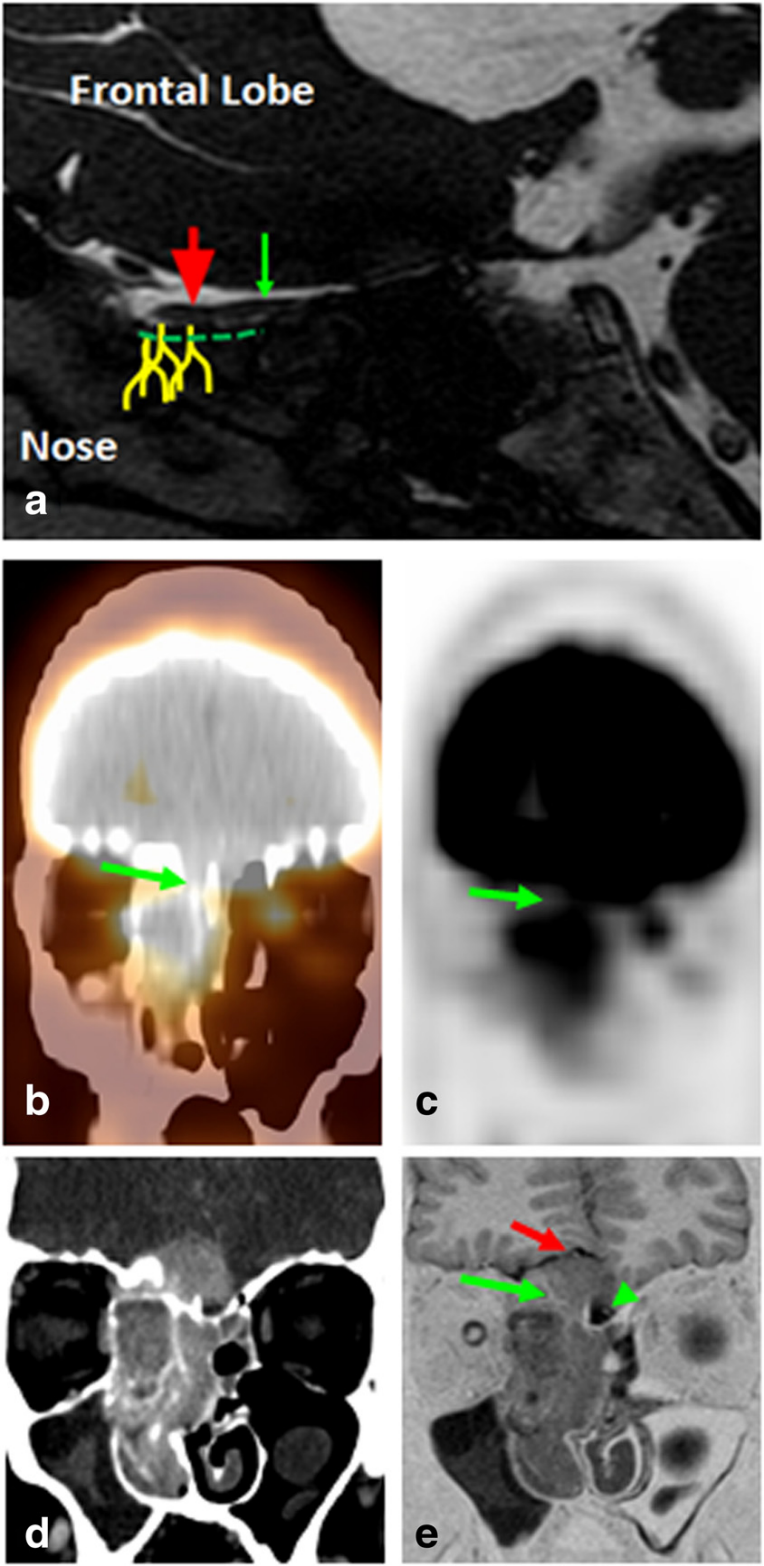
a Sagittal steady state free precession (SSFP) MRI image of the brain showing the olfactory nerve anatomy: The fibers in the superior nasal mucosa (yellow fibers), ascend through the fenestrated cribriform plate of the ethmoid bone (green dashed line) to reach the anteriorcranial fossa and continue as the olfactory bulb (red arrow) and tract (green arrow) coursing atthe inferior surface of the ipsilateral frontal lobe. b–e A 38-year-old male presented with a 6month history of anosmia and episodic epiphoresis, nasal stuffiness. Coronal fused (a) andunfused (b) PET/CT images demonstrate a large aggressive hypermetabolic mass centered on thesuperior aspect of the nasal cavity, extending superiorly into the right anterior cranial fossa andcribriform plate of ethmoid bone, involving the expected location of the right olfactorynerve/bulb/track (arrow). Our differential diagnosis was olfactory neuroblastoma (Esthesioneuroblastoma), or sinonasal carcinoma/melanoma. Coronal contrast enhanced CT (c) demonstrate the enhancing mass extending into the anterior cranial fossa at the expected locationof CNI. Coronal short tau inversion recovery (STIR) (e) images confirm the intracranial extension (arrow) with involvement of the right olfactory nerve/bulb (red arrow). Note the normal left olfactory track (green arrowhead). Surgical pathology showed an olfactory neuroblastom
